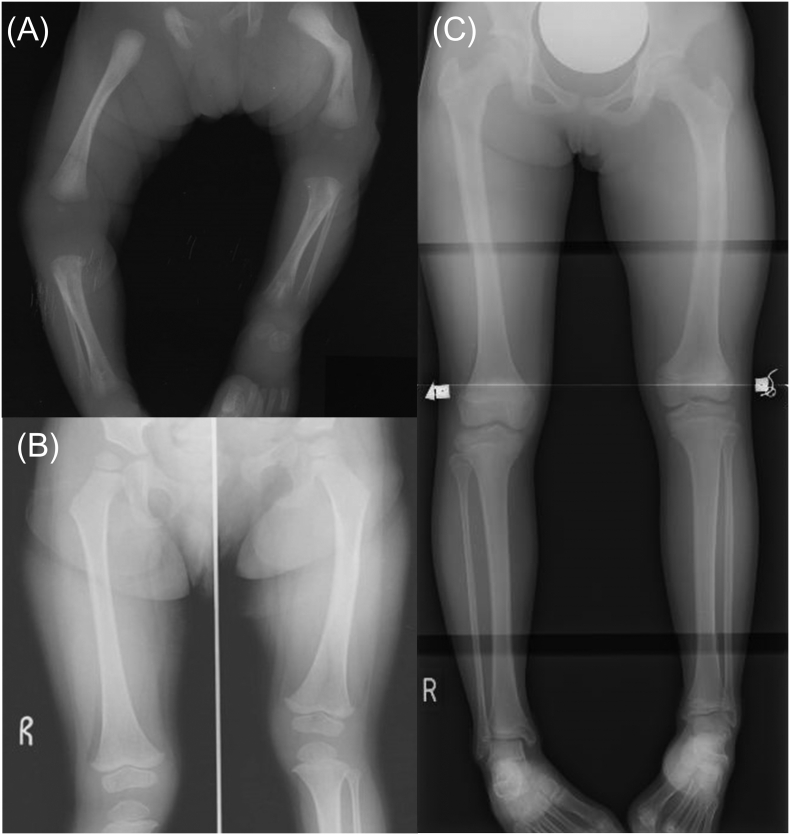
X-rays of the lower limb at birth (A), at two years of age (B), and at ten years of age (C). (A) Shortening and curvature of the left leg was observed at birth. (B, C) Discrepancy in leg length appeared at two years of age (B) and remained at ten years of age (C).
